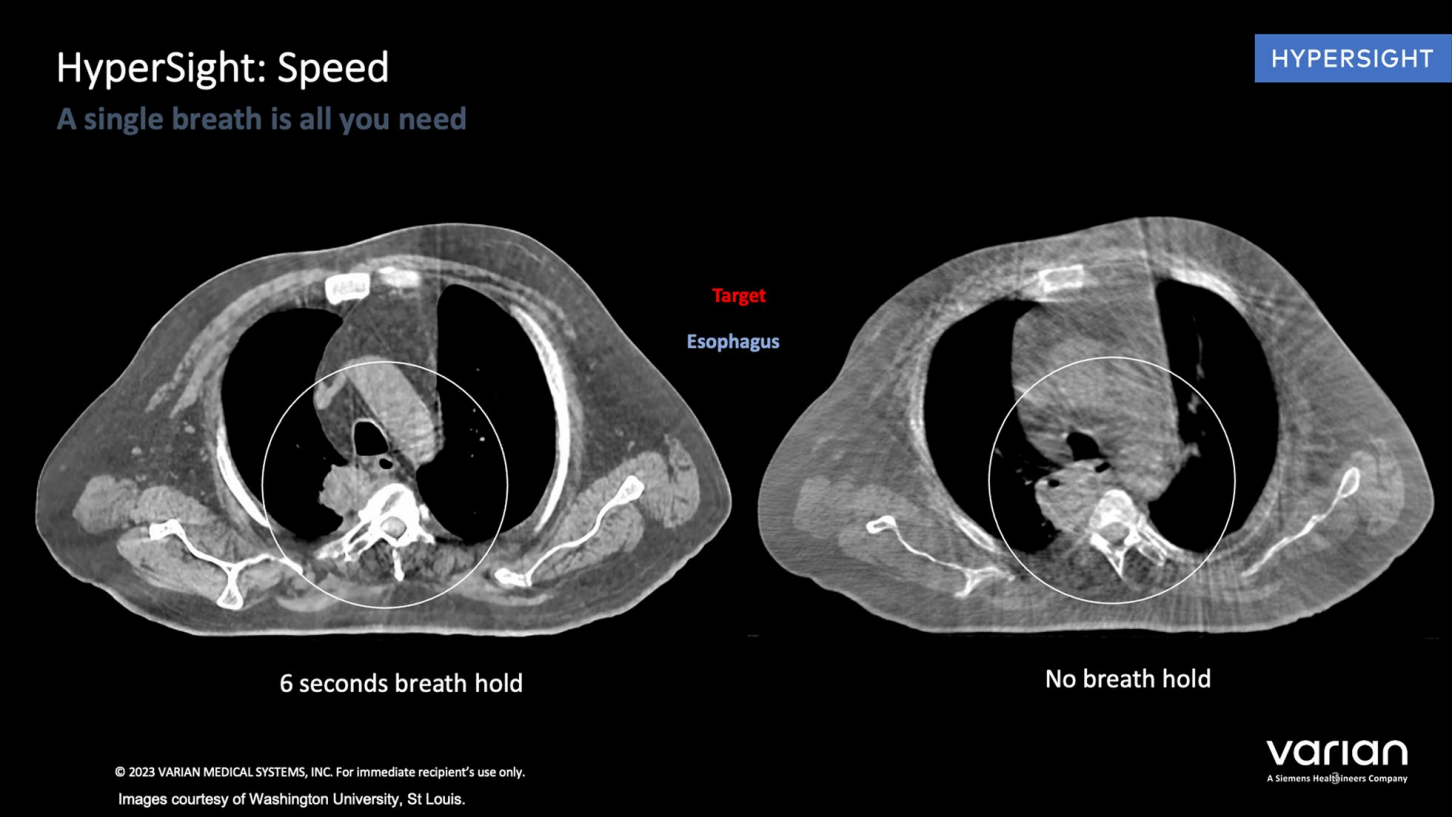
HyperSight™ CBCT technology captures larger images with better contrast within only 6 s, which allows tumors that move with respiration to comfortably perform a single breath-hold for the machine to obtain high-quality images needed for daily treatment planning and delivery
